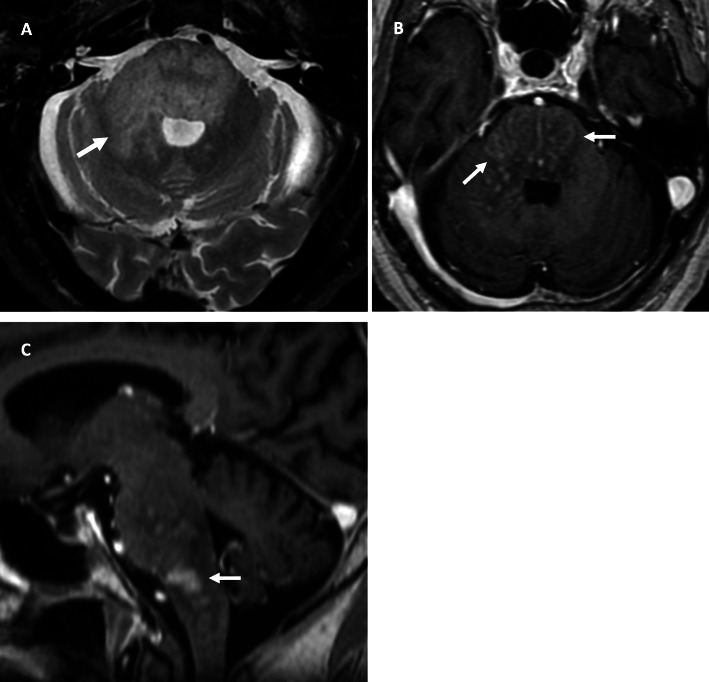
MRI brain at initial presentation. a Axial T2-weighted image of the brainstem shows diffuse signal change within the pons and right middle cerebellar peduncle (arrow). b Axial T1-weighted post-contrast image showing punctate and linear areas of enhancement (arrows) in the pons and right cerebellar peduncle. c Sagittal T1-weighted post-contrast image shows patchy enhancement in the pons and pontomedullary junction
